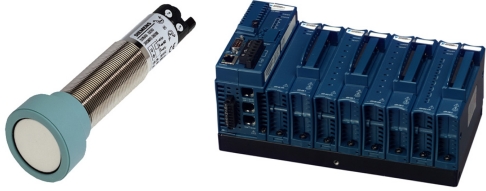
Ultrasonic sensor used in this work (left) model Sonar Bero PXS400 M30 K3 (Siemens AG, Munich, Germany). Electronic device for data acquisition and control of the trials (right) model compactFieldPoint 2120 (National Instruments, Austin, TX, USA).
